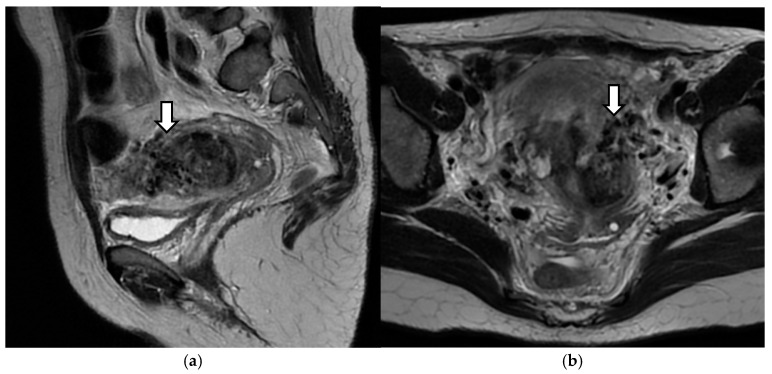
Dynamic magnetic resonance images of the pelvis. Arrows show numerous signal voids in the uterine wall: (a) longitudinal section; (b) transverse section.
